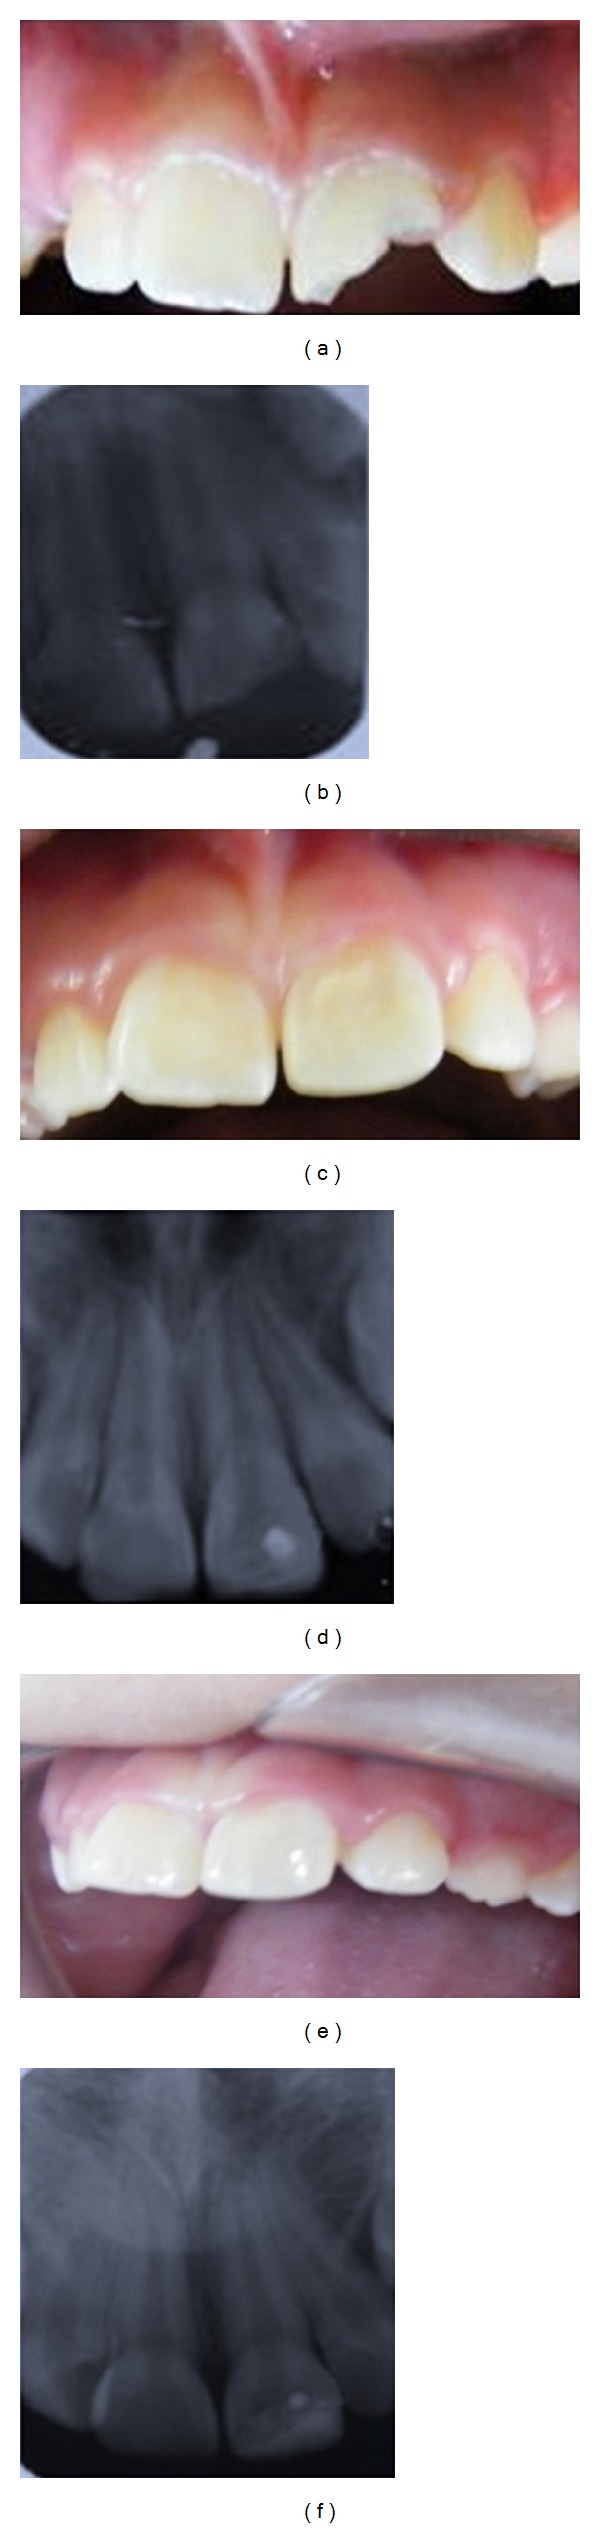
(a) Initial clinical image of patient 17 hours after trauma with complicated crown fracture in the maxillary left central incisor. (b) Initial radiograph showing loss of dental structure, complete root development, closed apices, and absence of root or alveolar bone fractures. (c) Resin reconstruction after treatment. (d) Posttreatment radiograph showing partial pulpotomy with resin reconstruction. (e-f) Clinical and radiographic images 25 months after treatment. Patient's teeth were found to be vital; no pain to percussion or palpation, with functional restorations.
